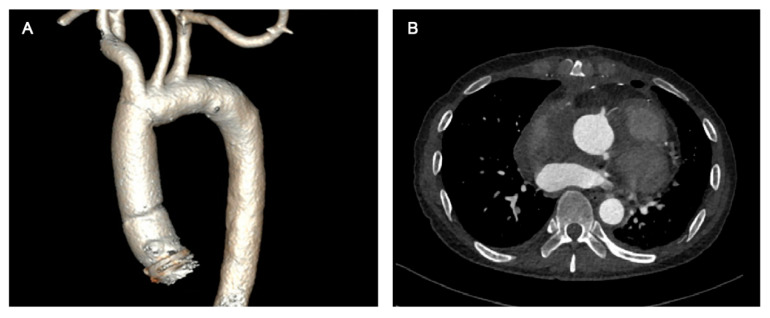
Postoperative aortic CTA scan revealed no leakage around the coronary ostia and an absence of pseudoaneurysm. (A) Three-dimensional reconstruction of aortic CTA; (B) Aortic CTA image at the coronary ostial level. CTA: computed tomographic angiography.
